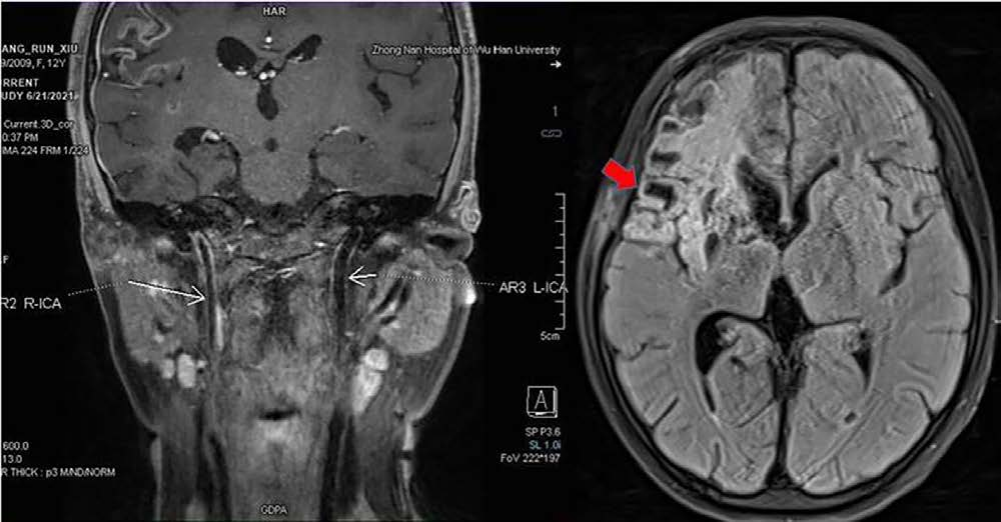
Two months after the first clipping operation, MRI showed stenosis of the right internal carotid artery and white matter damage in right frontal lobe. MRI = magnetic resonance imaging.
